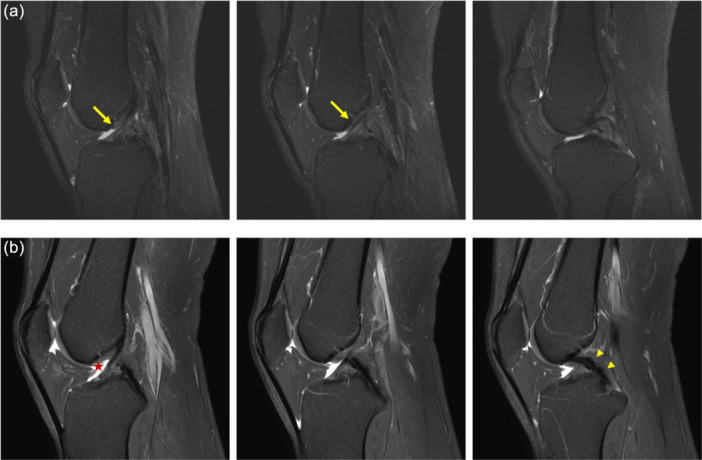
Sagittal fat‐suppressed T2‐weighted MR images of a single patient at two time points. (a) MR images obtained prior to PCL injury: the ACL is in close contact with the roof of the intercondylar notch; the PCL is intact with a normal course. (b) MR images acquired after partial PCL injury: the ‘empty roof sign’ is now positive (★); the PCL appears continuous (►), but compared with the previous examination, a subtle alteration in its course is evident. ACL, anterior cruciate ligament; MR, magnetic resonance; PCL, posterior cruciate ligament.
